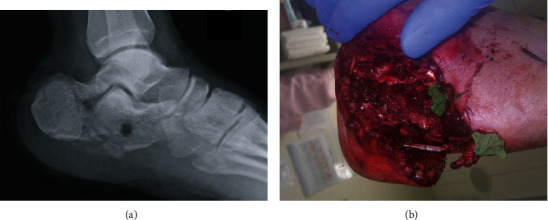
(a) Lateral view of the initial radiograph revealed a tongue-type calcaneus fracture. (b) Severe contamination by standing water of a 10 cm wound on the lateral side of the calcaneus; small leaves were attached to the wound.
